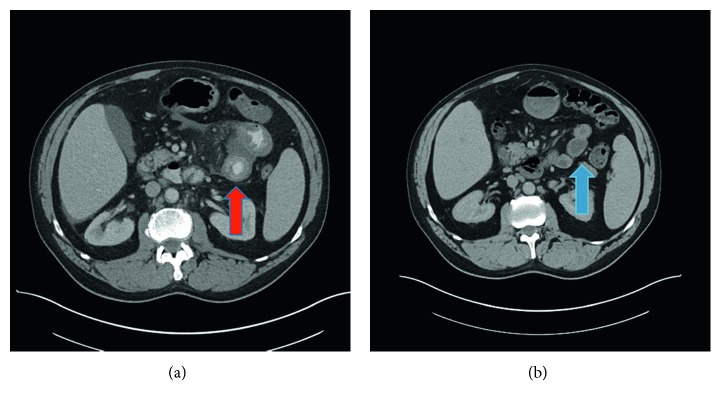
CT abdomen findings before and after treatment. (a) Before treatment, the patient was noted to have jejunal enteritis, which is indicated by the target sign (red arrow). (b) After treatment, the patient had resolution of jejunitis (blue arrow).
